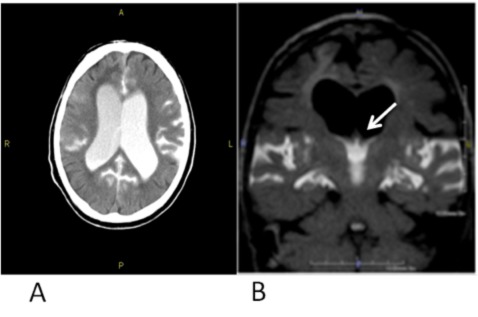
Patient with communicating hydrocephalus. Metrizamide CT cisternography indicates ventricular reflux (black arrow) (A). However, cerebrospinal fluid (CSF) flow into the lateral ventricles from the third ventricle is not observed using the Time-SLIP method (white arrow) in the hydrocephalic brain (B). Supplementary video 3.
